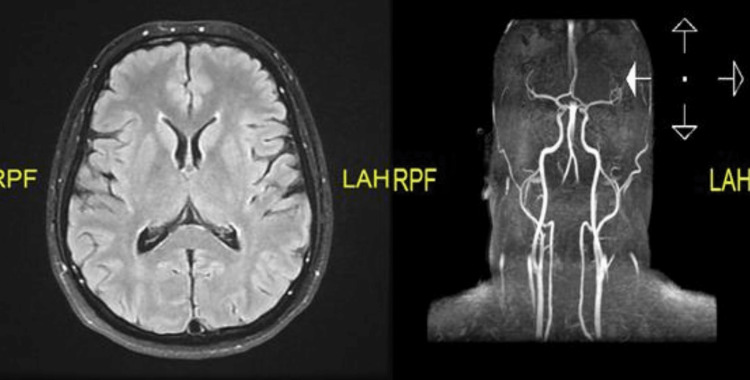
Axial FLAIR MRI of the brain at the level of the basal ganglia and MRA image showing normal findings FLAIR: fluid-attenuated inversion recovery; MRA: magnetic resonance angiography.
